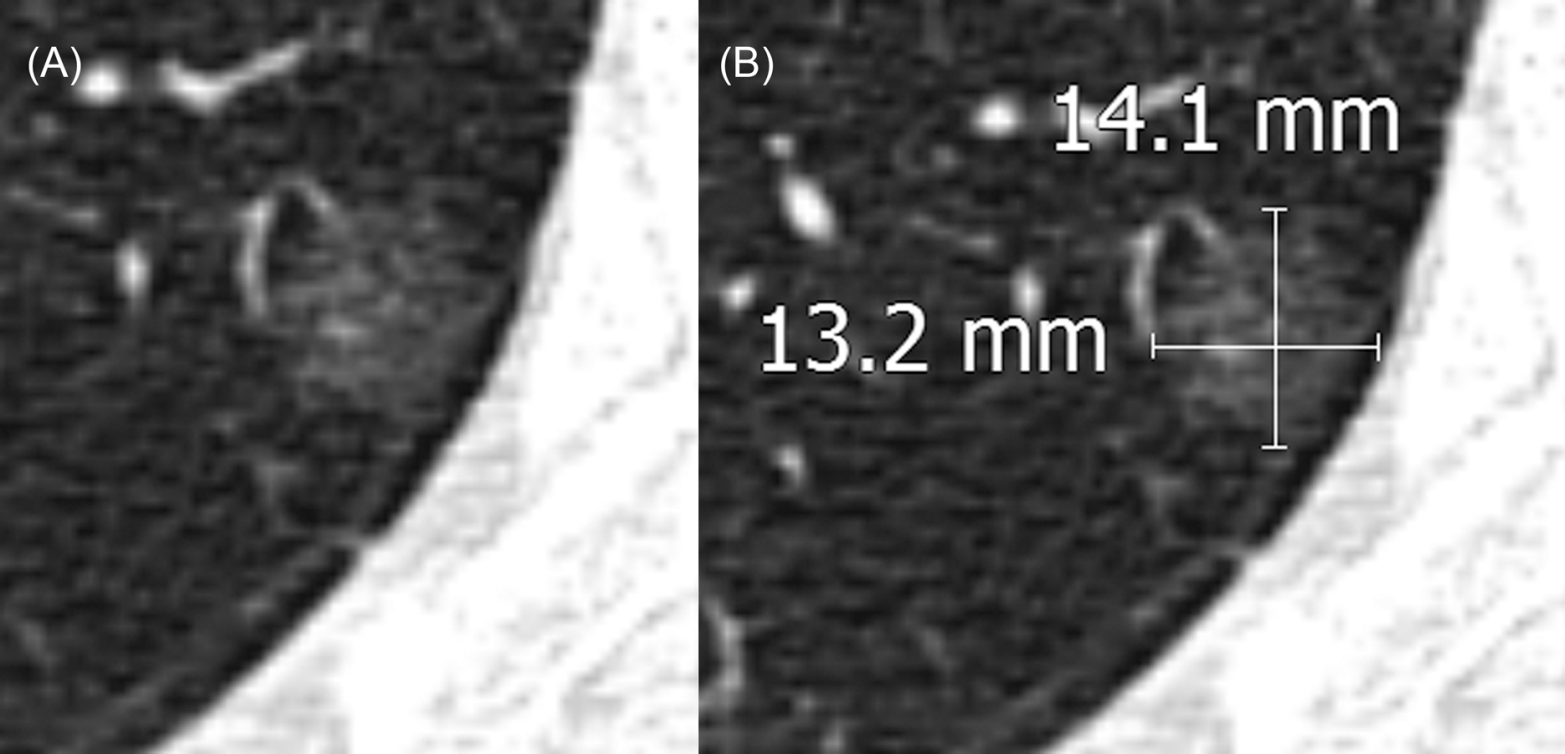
A pure ground-glass nodule in the right upper lobe of the lung in a 73 year-old female. (A) The subsolid nodule has a less distinct margin than the solid nodule. (B) The longest diameter along with its maximum perpendicular diameter was measured. Longest diameter measurement differences between scans ranged from -1.8 to 1.3 mm when the follow-up CT reader was different from that at initial CT.
